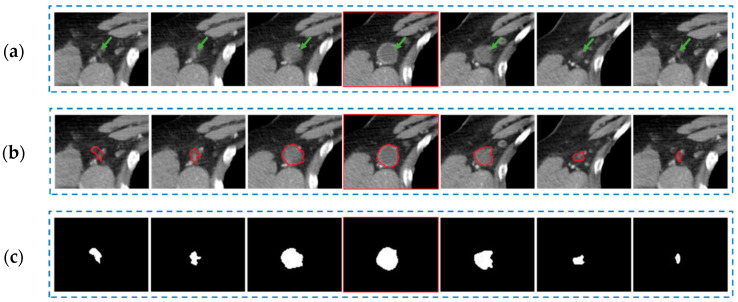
One example of the tumor segmentation process, in which the central slice is outlined with red color. (a) Identified tumor on the CT slices. (b) Tumors outlined using our developed CAD software. (c) Segmented tumor masks.
